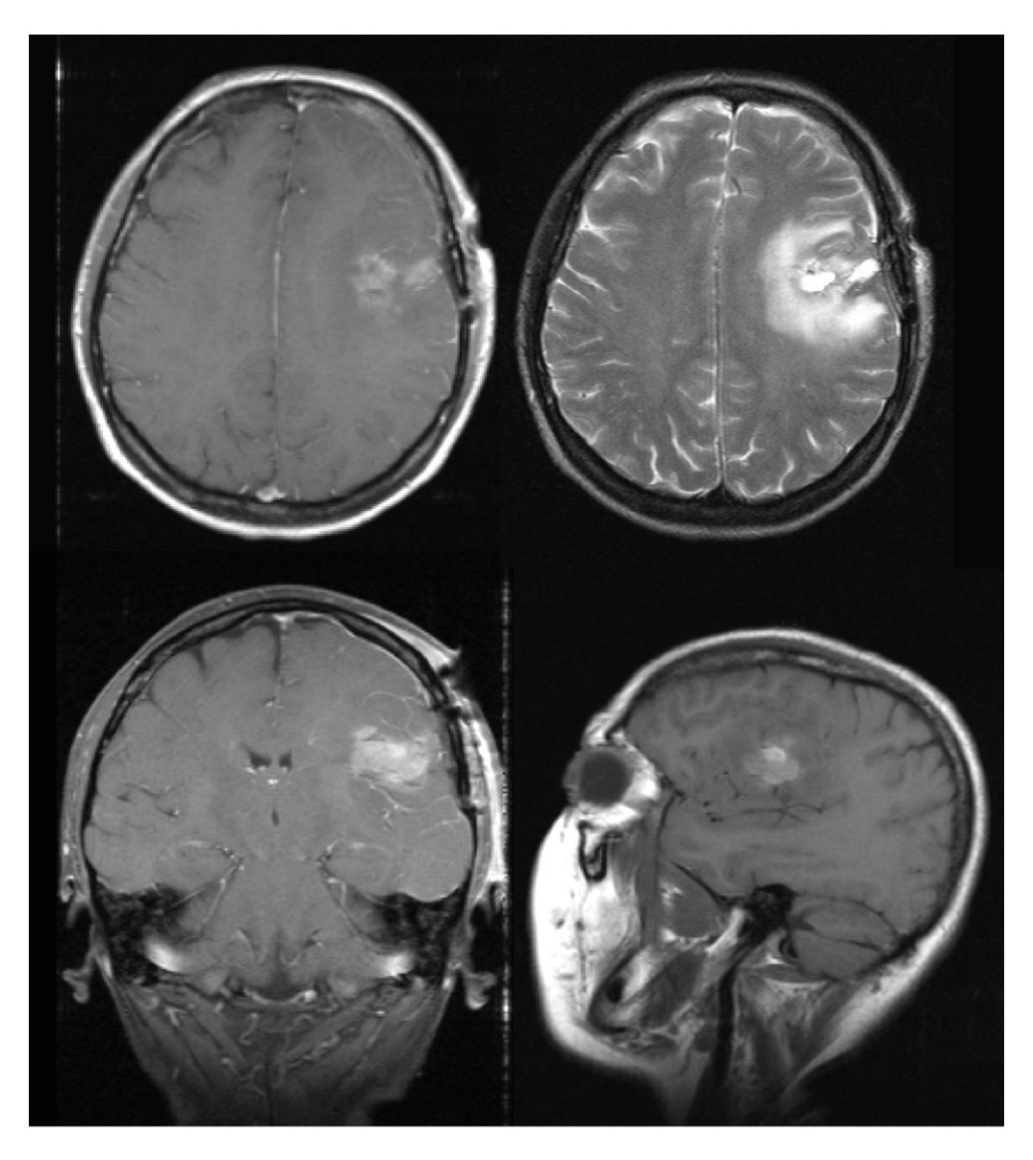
Postoperative Brain MRI Brain MRI after tumor excision showing post-operative changes in the left frontotemporal region
